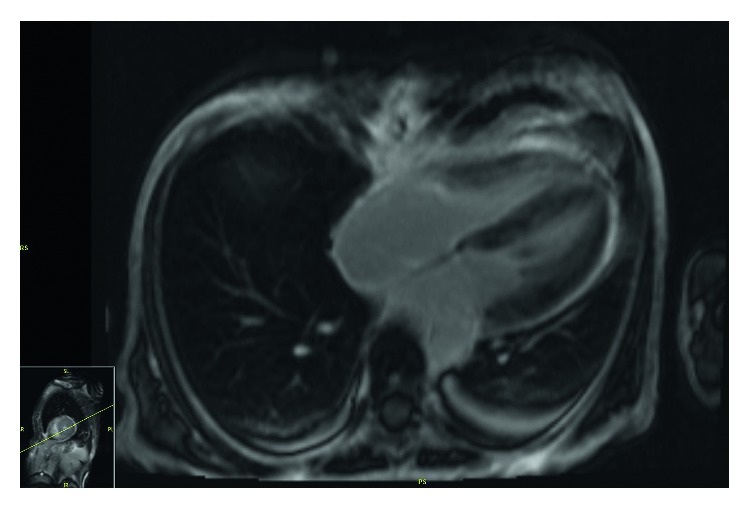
Cardiac MRI. Evidence of patchy focal late gadolinium enhancement of the pericardium with suboptimal nulling of the myocardium.
